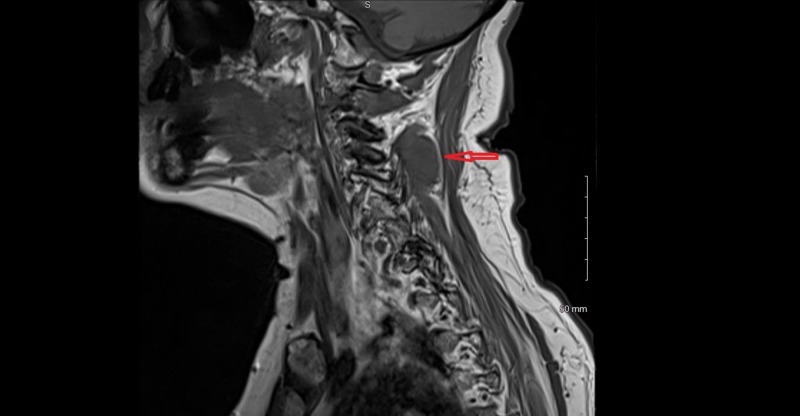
Parasagittal magnetic resonance imaging (MRI) of lesion. T1 weighted MRI of cervical spine with parasagittal image showing a hypointense mass in posterior elements from C2 to C4 measuring approximately 3.5 cm x 1.7 cm x 1.6 cm.
